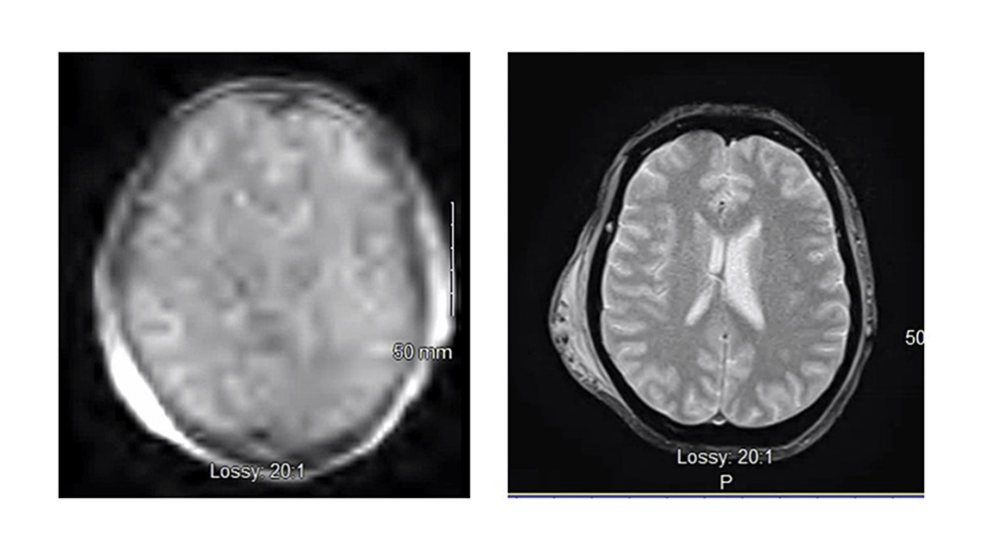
T2 axial MRI. Left - pMRI; right - traditional T2 MRI of the same patient, status post-trauma with associated extracalvarial hematoma. MRI, magnetic resonance imaging; pMRI, portable magnetic resonance imaging
